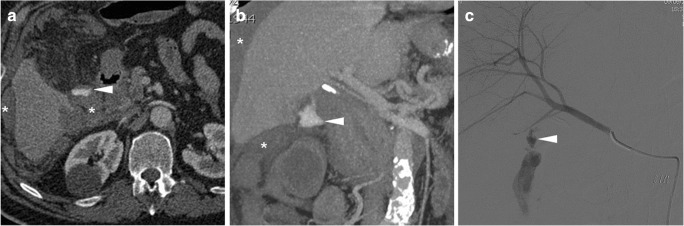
Active haemorrhage diagnosed 48 h after laparoscopic cholecystectomy as extravascular contrast “blush” (*arrowheads*) on arterial (a) and portal venous (b) CT images, within infrahepatic haematoma (*), which was confirmed angiographically (c) and effectively treated by embolisation
